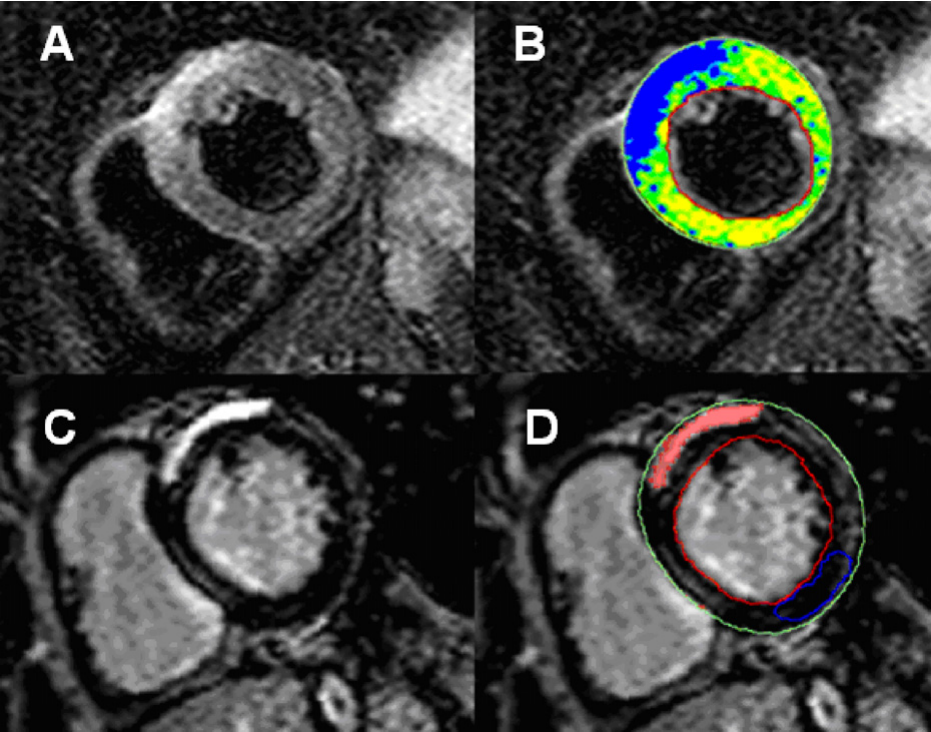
Myocardial edema in a patient with active myocarditis. A: T2-weighted CMR image showing subepicardial edema in the anteroseptal segment. B: Computer-aided signal intensity analysis of the T2-weighted image with color-coded display of relative signal intensity, normalized to skeletal muscle. Blue indicates a signal intensity ratio of myocardium/skeletal muscle of ≥2.0, indicating edema, green indicates normal signal intensity (1.4-1.9). C: Contrast-enhanced image (late gadolinium enhancement) showing a high signal intensity in the same region indicating necrosis. D: Computer-aided signal intensity analysis of the necrosis image with color-coded display of relative signal intensity, normalized to remote myocardium. Red indicates a signal intensity of >5 standard deviations above remote myocardium.
